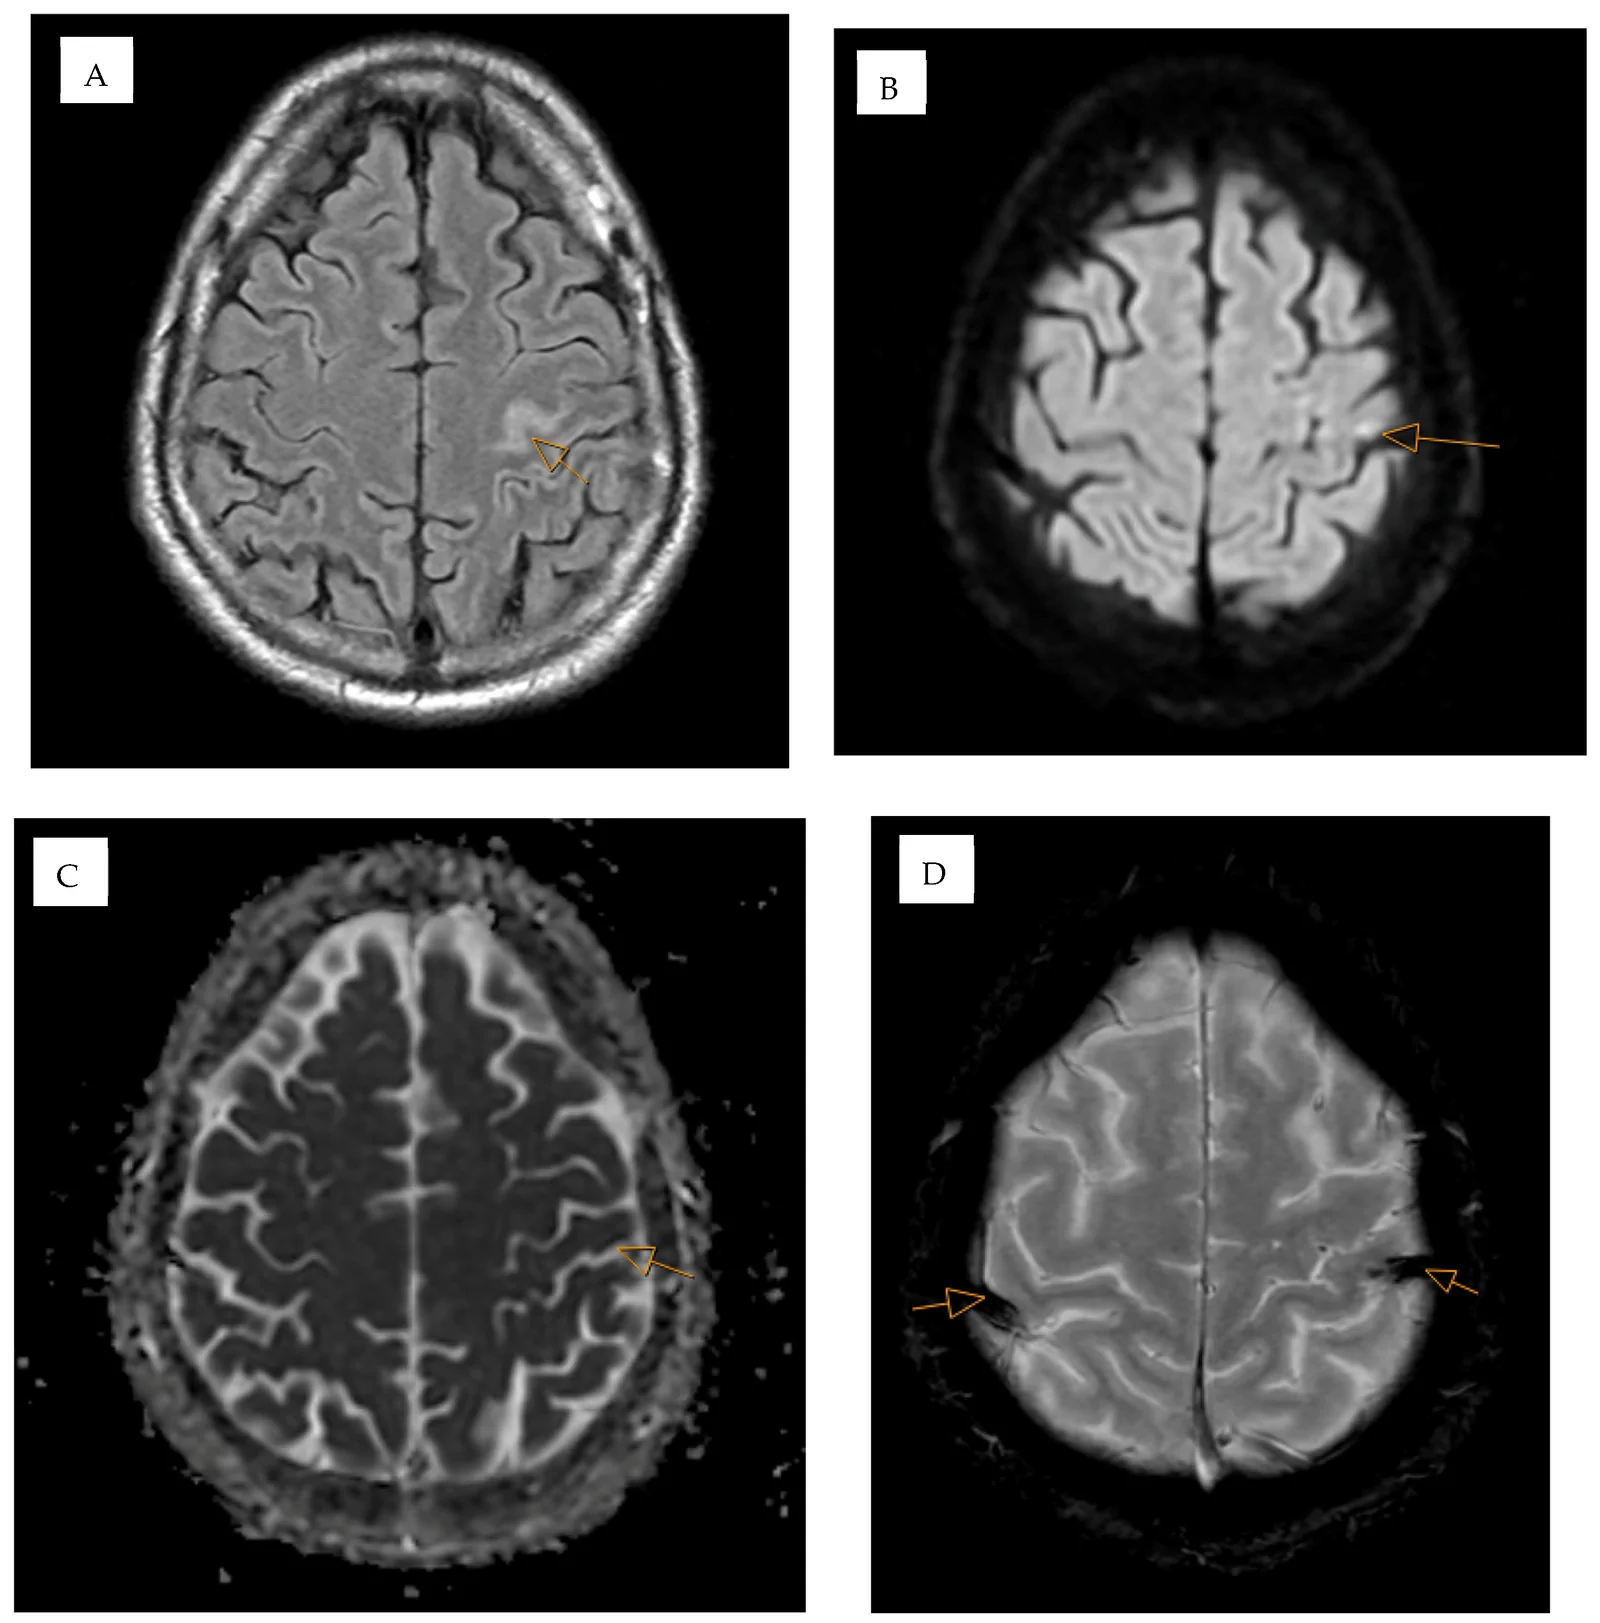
Multimodal MRI of the brain. (A) Axial fluid-attenuated inversion recovery (FLAIR) image demonstrating focal cortical hyperintensity in the left superior frontoparietal region (arrow). (B) Diffusion-weighted imaging (DWI) demonstrating corresponding cortical hyperintensity (arrow). (C) Apparent diffusion coefficient (ADC) map demonstrating corresponding cortical hypointensity (arrow). (D) Gradient-recalled echo (GRE) image demonstrating susceptibility within bilateral cortical veins (arrows), more pronounced on the left.
